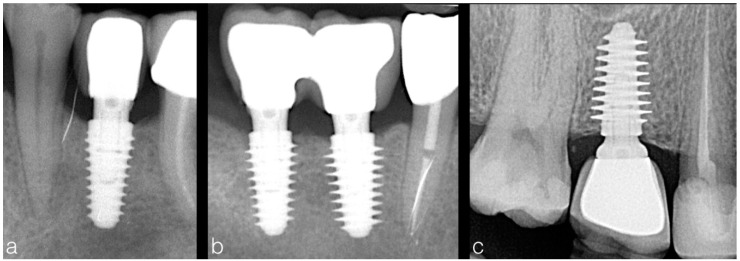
One year post-loading periapical radiographs showing implants placed in different bone density with variable thread depth (a—4 mm, b—4.5 and 5 mm, c—5.5 mm). Implants inserted in hard bone presented minimal thread depth (a) to avoid excessive bone compression, while implants with deep threads were used in soft bone (c) in order to attain higher primary stability.
